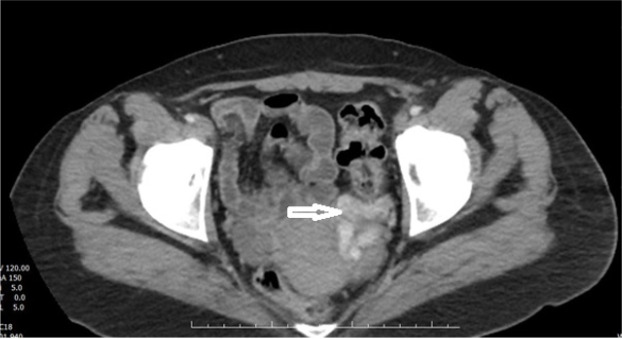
Axial view of intravenus contrast-enhanced abdominopelvic computed tomography scan demonstrates dilatation in the left gonadal vein and dilated vascular structures (white arrow).
